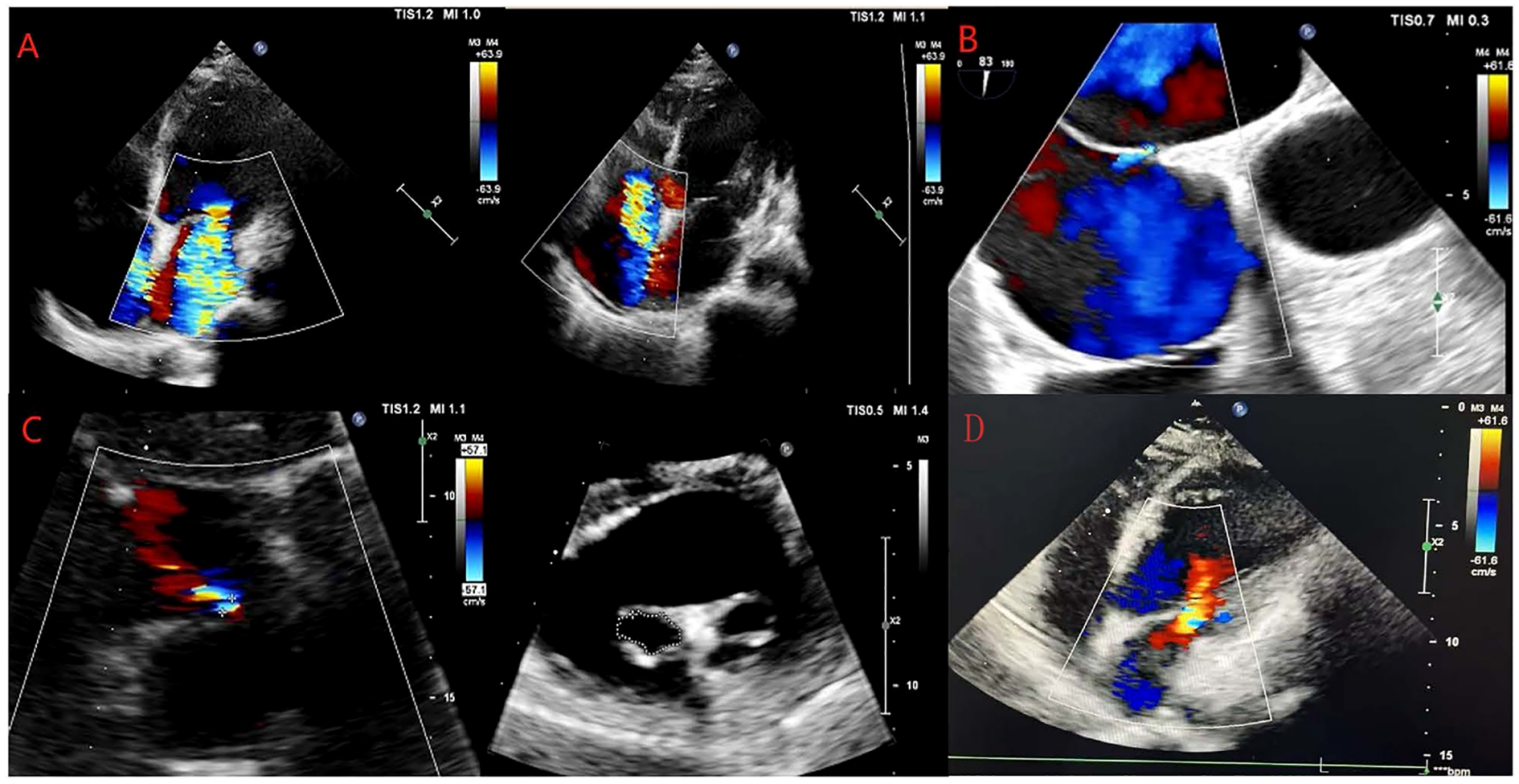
Changes in echocardiography examinations of the patient. (A–D) show the results of the echocardiography examinations of the patient on May 31, 2024, June 11, 2024, June 24, 2024, and February 14, 2025.
